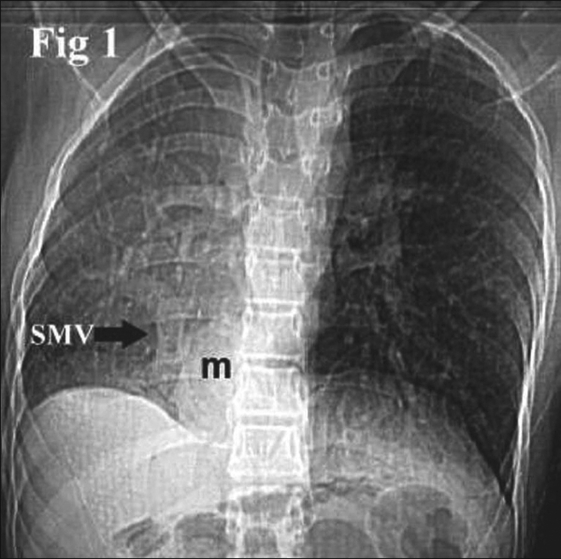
A frontal chest radiograph of a 15-year-old Saudi girl with scimitar syndrome, demonstrating a scimitar sign (msv), focal herniation of liver (m) mimicking a right paraspinal mass or sequestration, slightly opaque and small right hemithorax with nonvisualization of heart shadow
